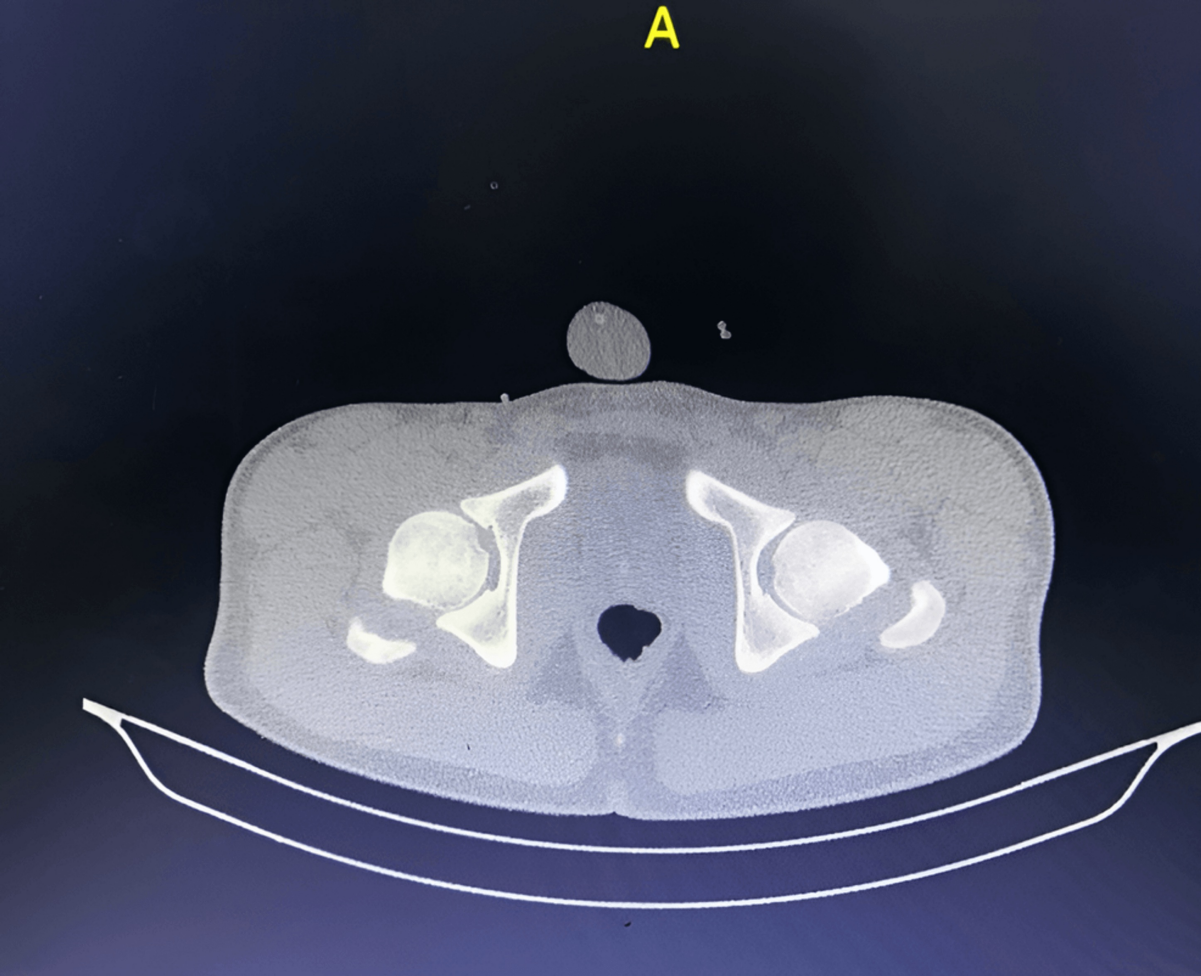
Axial computed tomography views after reduction showed relocation of the femoral head without fracture.
